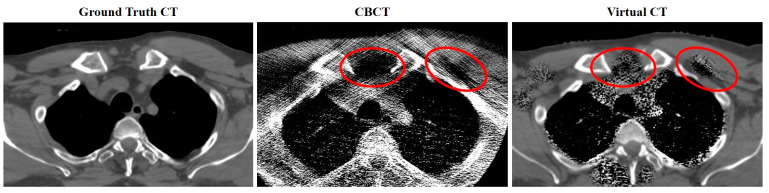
Demonstrating the effects of beam hardening and photon starvation artifacts between the shoulders. Areas circled in red demonstrate inaccurate tissue overrides in the virtual CT due to artifacts in the CBCT.
